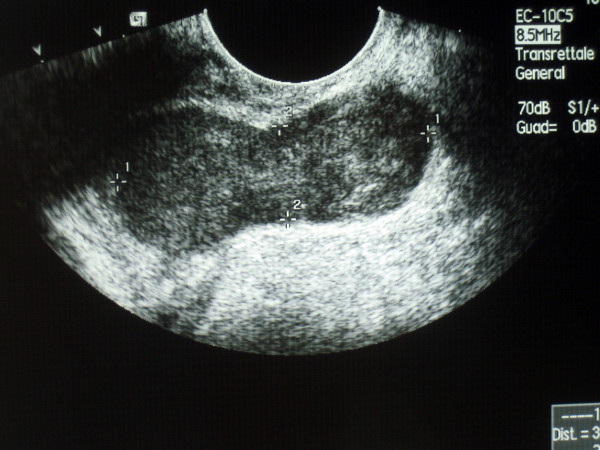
Endoanal ultrasonography (longitudinal plain) shows a bilobate, circumscribed 4 × 2 cm mass.
